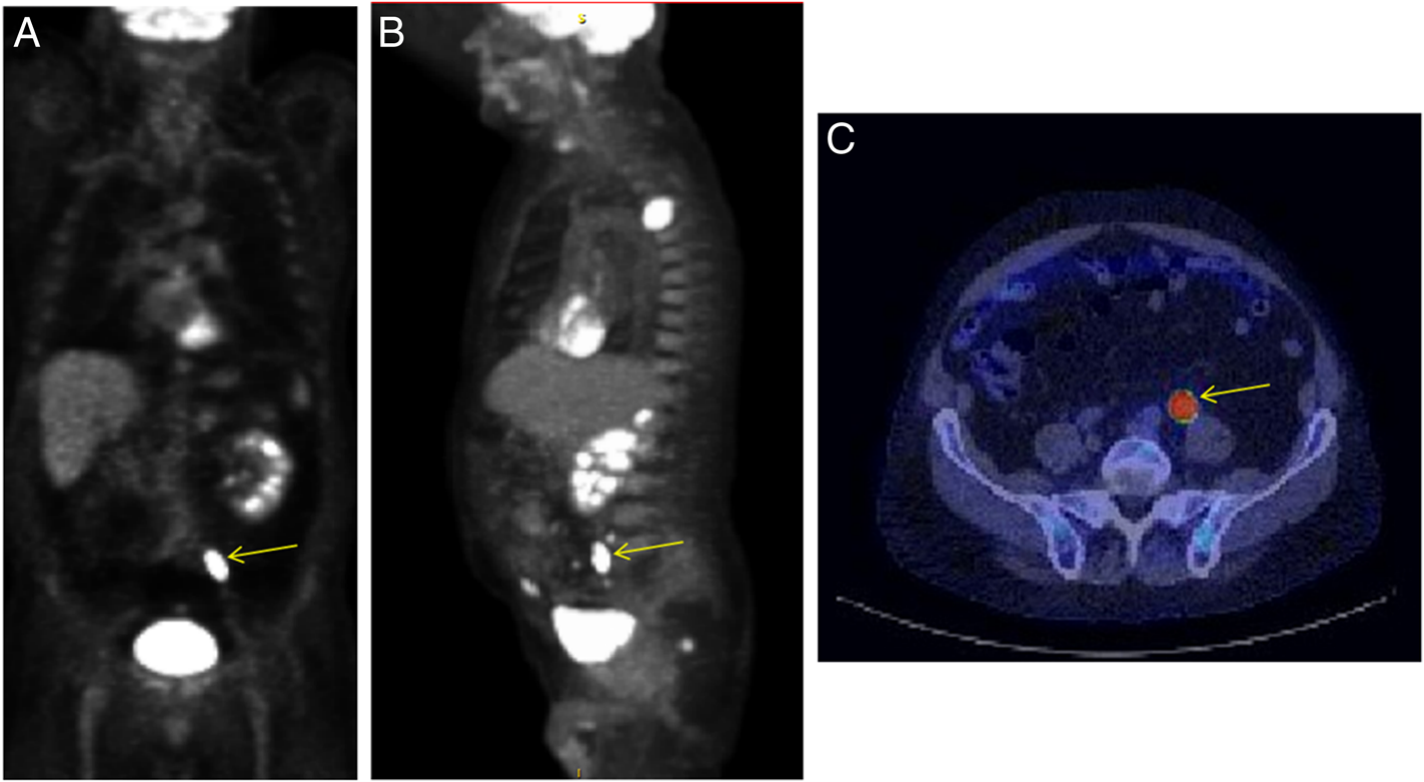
Focal [18 F]FDG uptake in a 79 year old male who was evaluated for an isolated right upper lobe pulmonary nodule. (a) coronal and (b) sagittal PET slices show indeterminate FDG avid foci (*arrows*) within the rectum. Area of increased uptake (*arrow*) was localized by PET/CT (c). Tissue biopsy revealed invasive moderately differentiated (grade 3 of 4) adenocarcinoma
